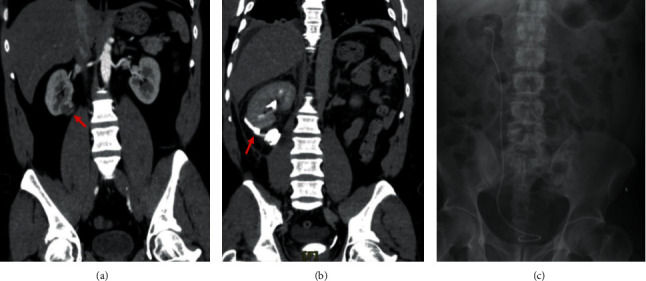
(a) Preoperative CT scan showing the lesion at the inferiomedial aspect of the right kidney. (b) Delayed phase CT scan demonstrating the urine leak from the site of partial nephrectomy. (c) Postoperative plain X-ray showing the JJ stent in position.
